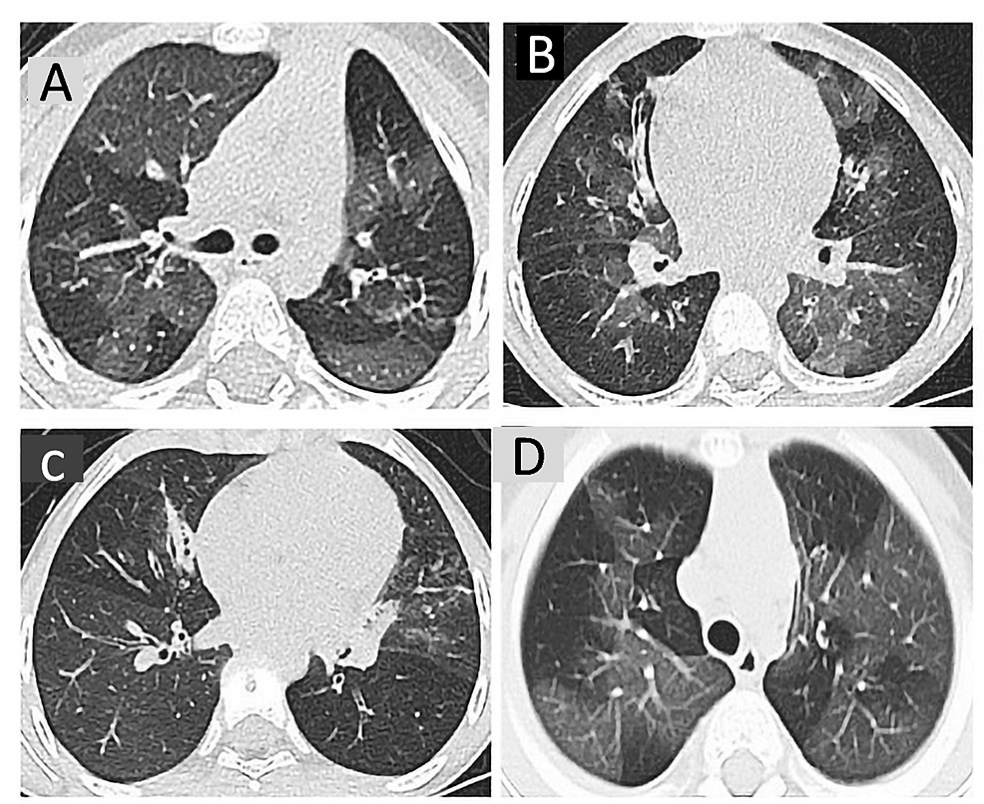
HRCT images from cases presented in Table 1 HRCT images A, B, and C correspond to bronchiolitis obliterans and primary ciliary dyskinesia cases 1-3 in Table 1 (previously reported cases in the medical literature). HRCT image D corresponds to case 4 in Table 1 (case presented in this manuscript) Images 2B and 2C were reprinted with permission from Guan et al. [5] HRCT: high-resolution computed tomography
